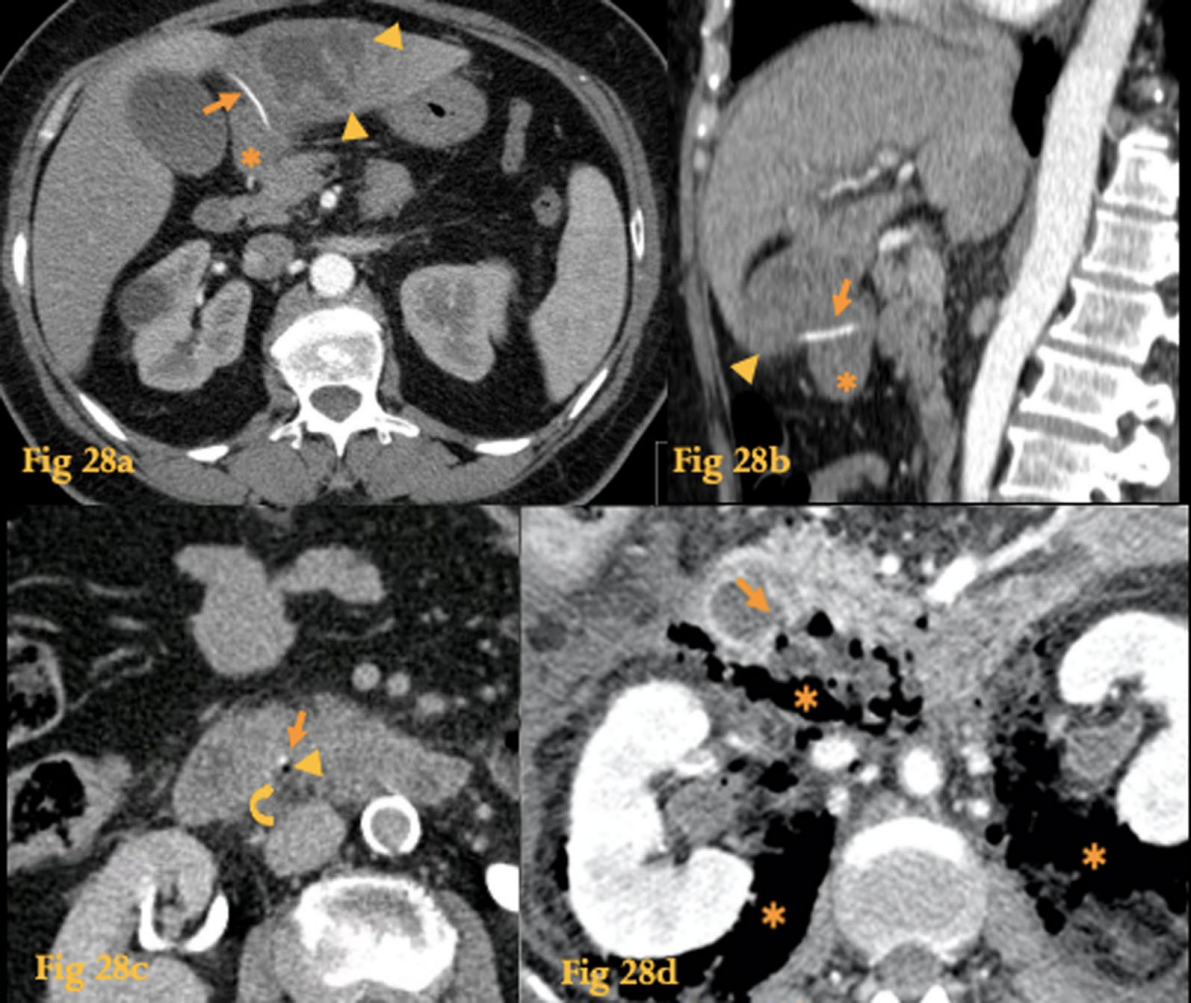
Rupture. Abdominal CT (a, b) of a patient who had ingested a fishbone (arrow). It perforated the duodenal bulb (asterisk) and lodged on the left lobe of the liver. Note the adjacent liver abscess (arrowheads) that formed as a result. CT of another patient (c) shows an endoluminal calcic density structure—fishbone (arrow) at D3, with a small amount of adjacent extraluminal gas (arrowhead) and fat stranding (curved arrow), indicative of retroperitoneal rupture. CT of yet another patient who had underwent ERCP (d) shows a discontinuity of the posterior wall of D3 (arrow) and extensive pneumoretroperitoneum (asterisk)
